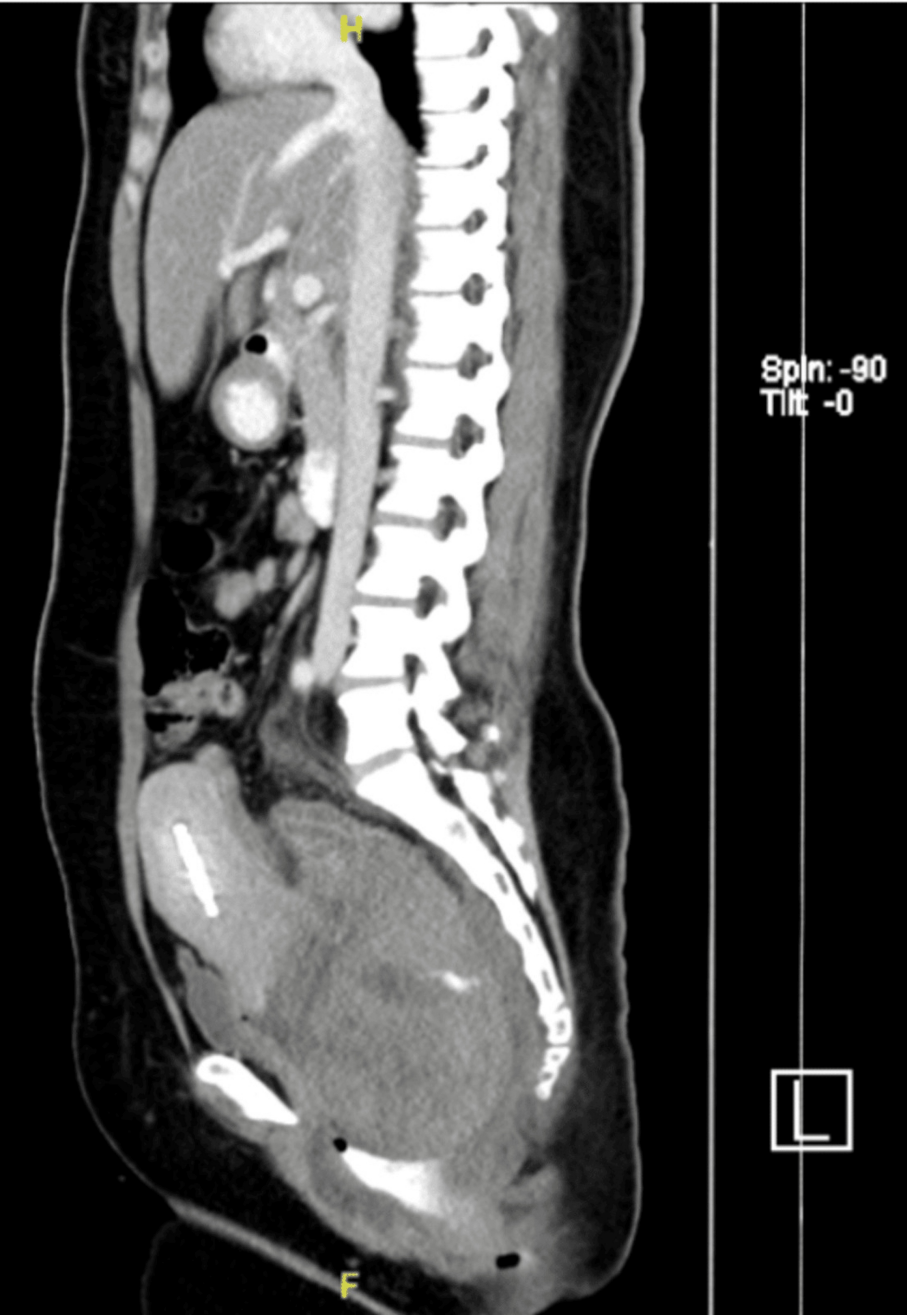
Sagittal contrast-enhanced computed tomography of the abdomen and pelvis showing a large intraluminal rectal mass initially interpreted as a rectal wall hematoma.
